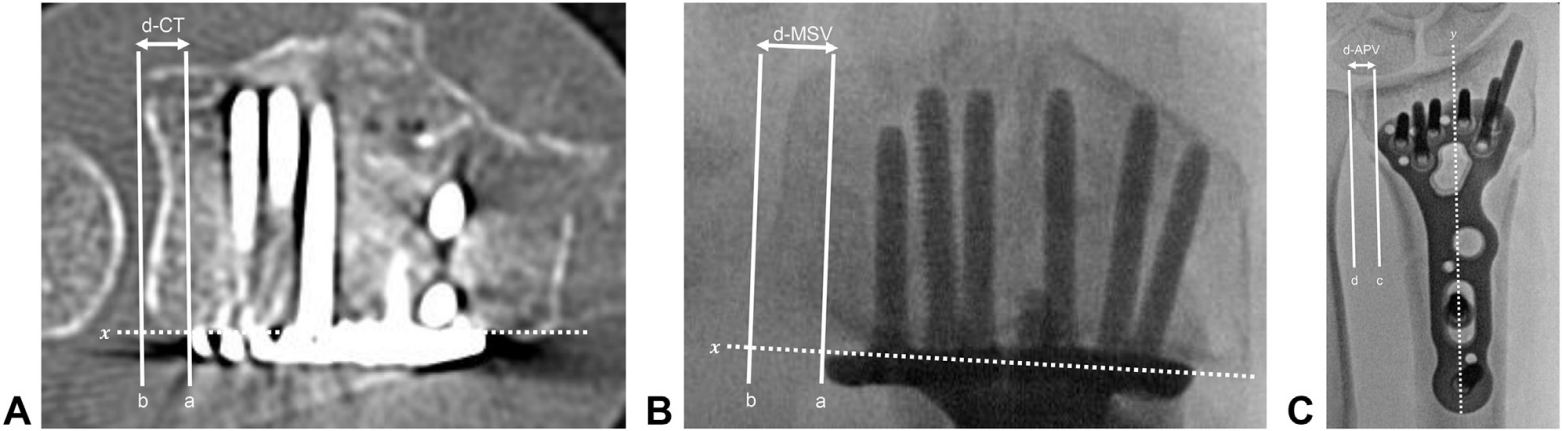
Measurement of d-values. A and B The white dotted line (x) in each image shows the line parallel to the volar edge of the distal radius. The distances between the 2 parallel lines are perpendicular to x, one of which is passing through the most ulnar edge of the volar locking plate (line “a”) and the other through the volar edge of the DRUJ (line “b”), were defined as d-CT and d-MSV, respectively. C The white dotted line (y) corresponds to the longitudinal axis of the radius. The distances between the 2 parallel lines perpendicular to y, one of which is passing through the most ulnar edge of the volar locking plate (line c) and the other through the volar edge of the DRUJ (line d), were defined as d-APV.
